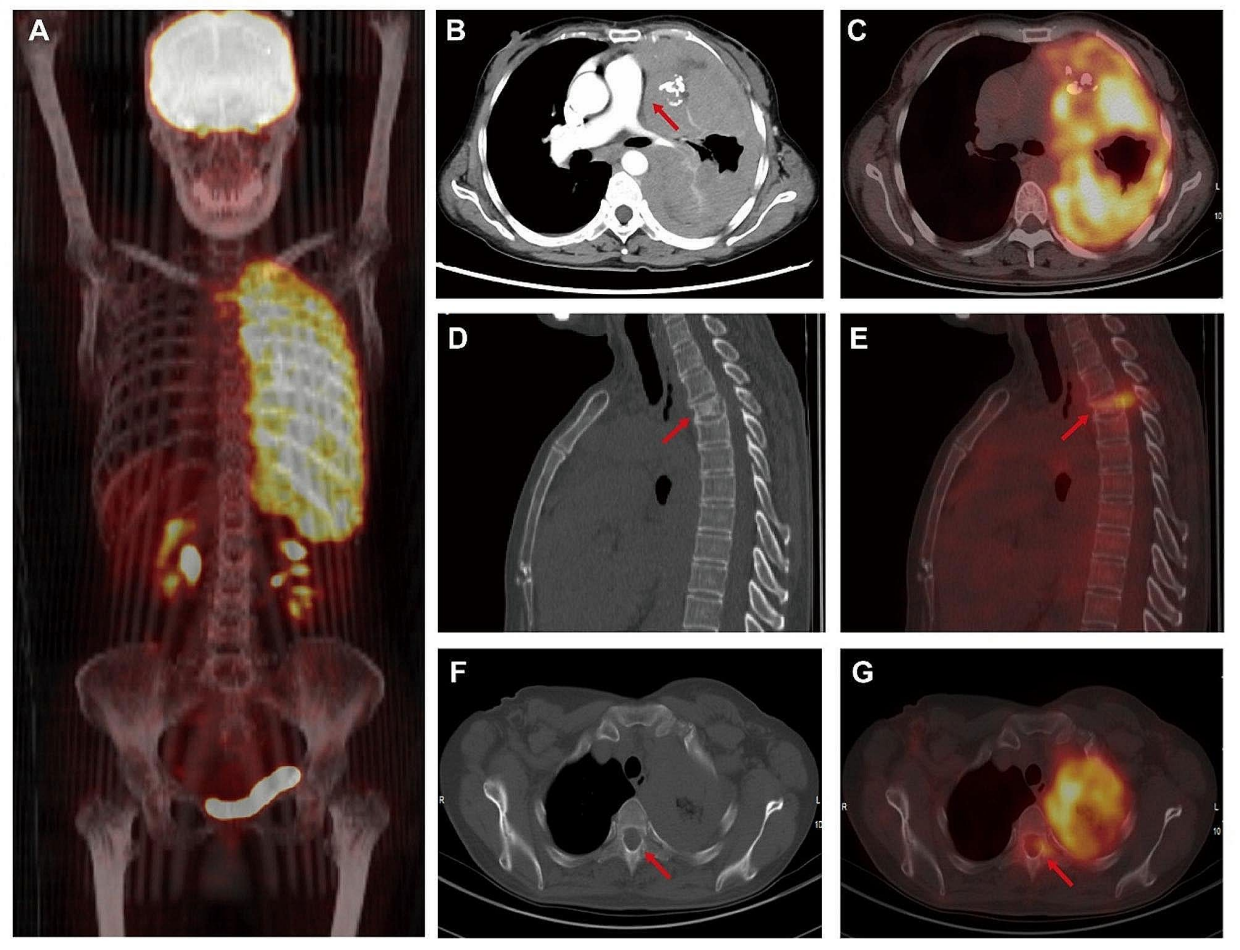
Radiographic imaging features of the chest. ^18^F-FDG PET/CT image showed increased ^18^F-FDG uptake of left pleura with a SUVmax of 8.3 (A and C). Contrast-enhanced CT image revealed the thickened pleura was mildly enhanced (B) and invading mediastinum and pericardium (arrow). A thoracic compression fracture was found in the second thoracic vertebrae (D, arrow) with a SUVmax of 8.2 (E, arrow). Osteolytic lesion of the second thoracic vertebrae attachment (F, arrow) was observed and it showed diffusely increased ^18^F-FDG uptake with a SUVmax 4.7 (G, arrow)
